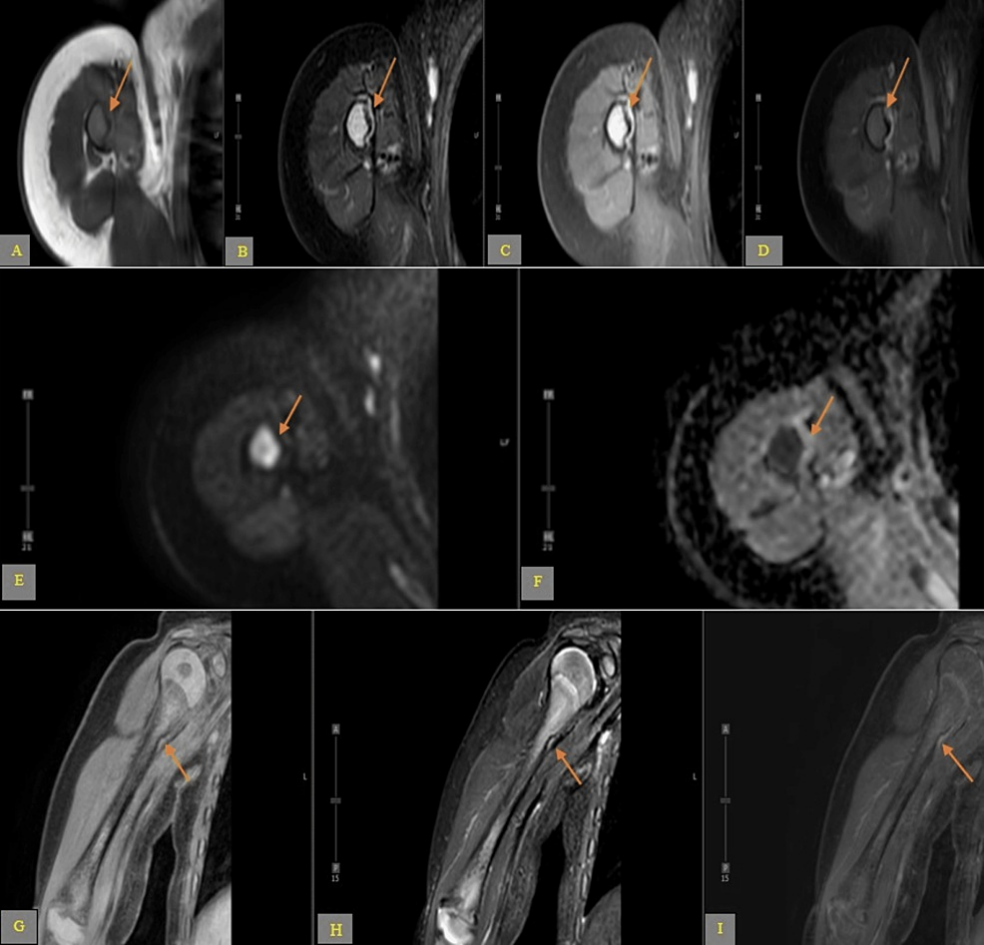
Series axial (A-F) and coronal (G-I) magnetic resonance imaging sequences. (A) T1 weighted imaging; (B) Fat saturated T2 weighted imaging; (C) Proton density; (D) T1-post contrast; (E) Diffusion weighted imaging; (F) Apparent diffusion coefficient; (G) Fat saturated T1 weighted imaging; (H) Short tau inversion recovery; (I) Post contrast subtraction sequence. These multiples magnetic resonance imaging sequences demonstrate a concave wavy appearing indentation of the medial meta-diaphysis cortex and periosteum of proximal right humerus with no discrete lesion, abnormal post-contrast enhancement, restriction diffusion, marrow, or soft tissue abnormality seen to correlate with the prior radiograph findings.
